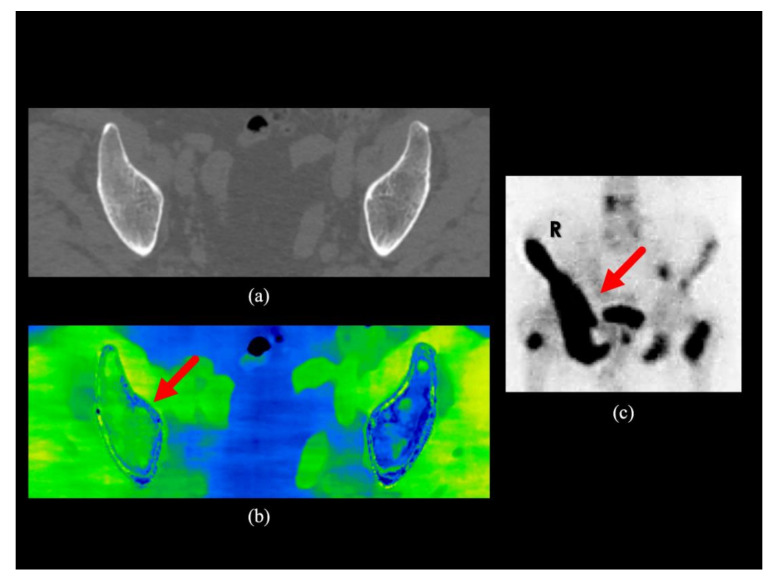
Clinical case 2. A man in his 70s with prostate cancer. (a) The transaxial image of the conventional CT of the pelvis does not indicate abnormal concentrations in the iliac bone; (b) There is an increase in water density compared to the contralateral side in the transaxial water-HAP image of the pelvis (red arrow); (c) The anterior view of the bone scintigraphy shows the right iliac bone metastases (red arrow). HAP; Hydroxyapatite.
